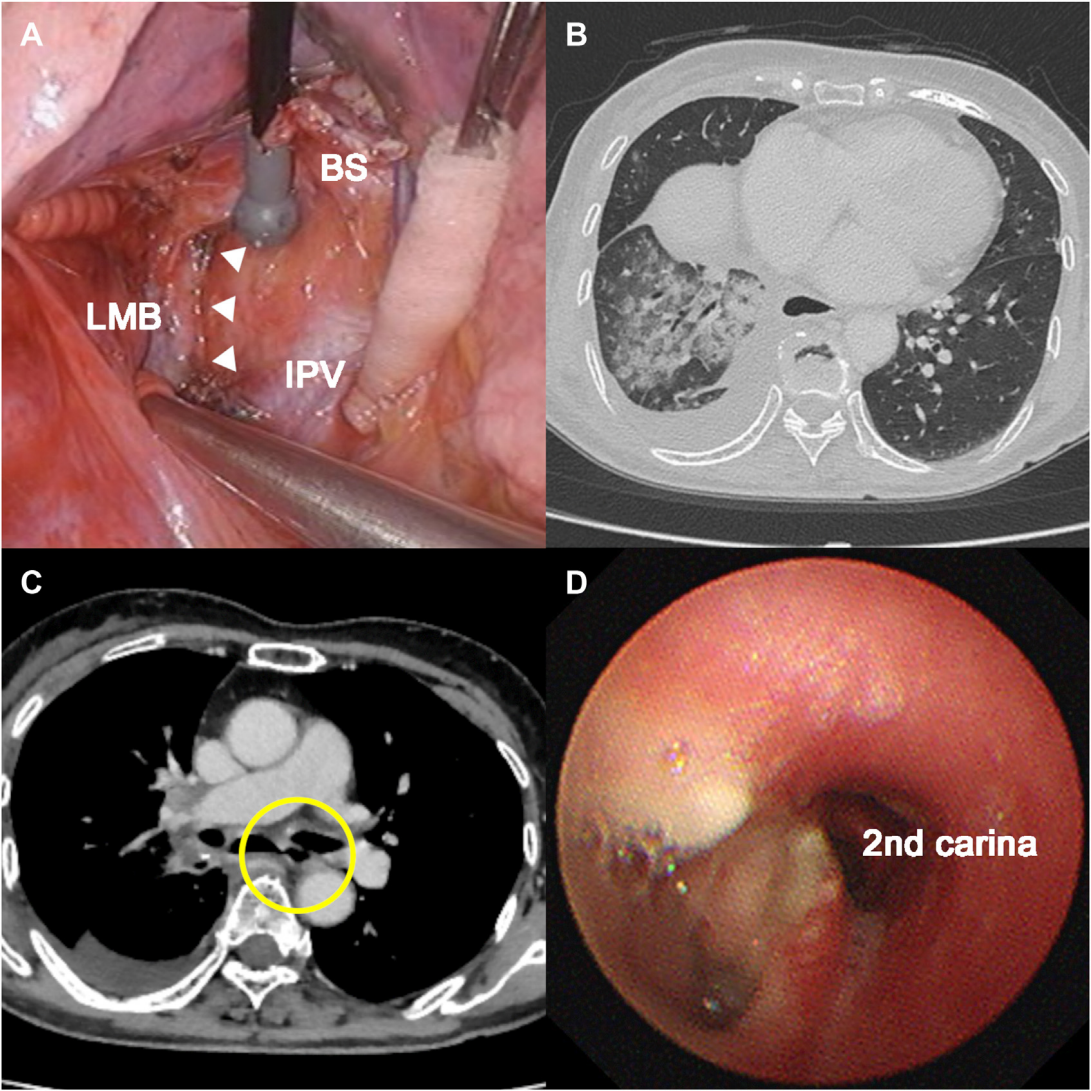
(A) Extensive systematic lymph node dissection of the mediastinum was performed (The arrowheads indicate the left main bronchus). Computed tomography of the thorax revealed (B) extensive infiltrative shadows centered around the right middle lobe without pneumothorax and (C) bronchoesophageal fistula between the left main bronchus and esophagus (circle). (D) Bronchoscopy revealed a small hole at the membranous portion of the left main bronchus. (BS, bronchial stump; IPV, inferior pulmonary vein; LMB, left main bronchus.)
